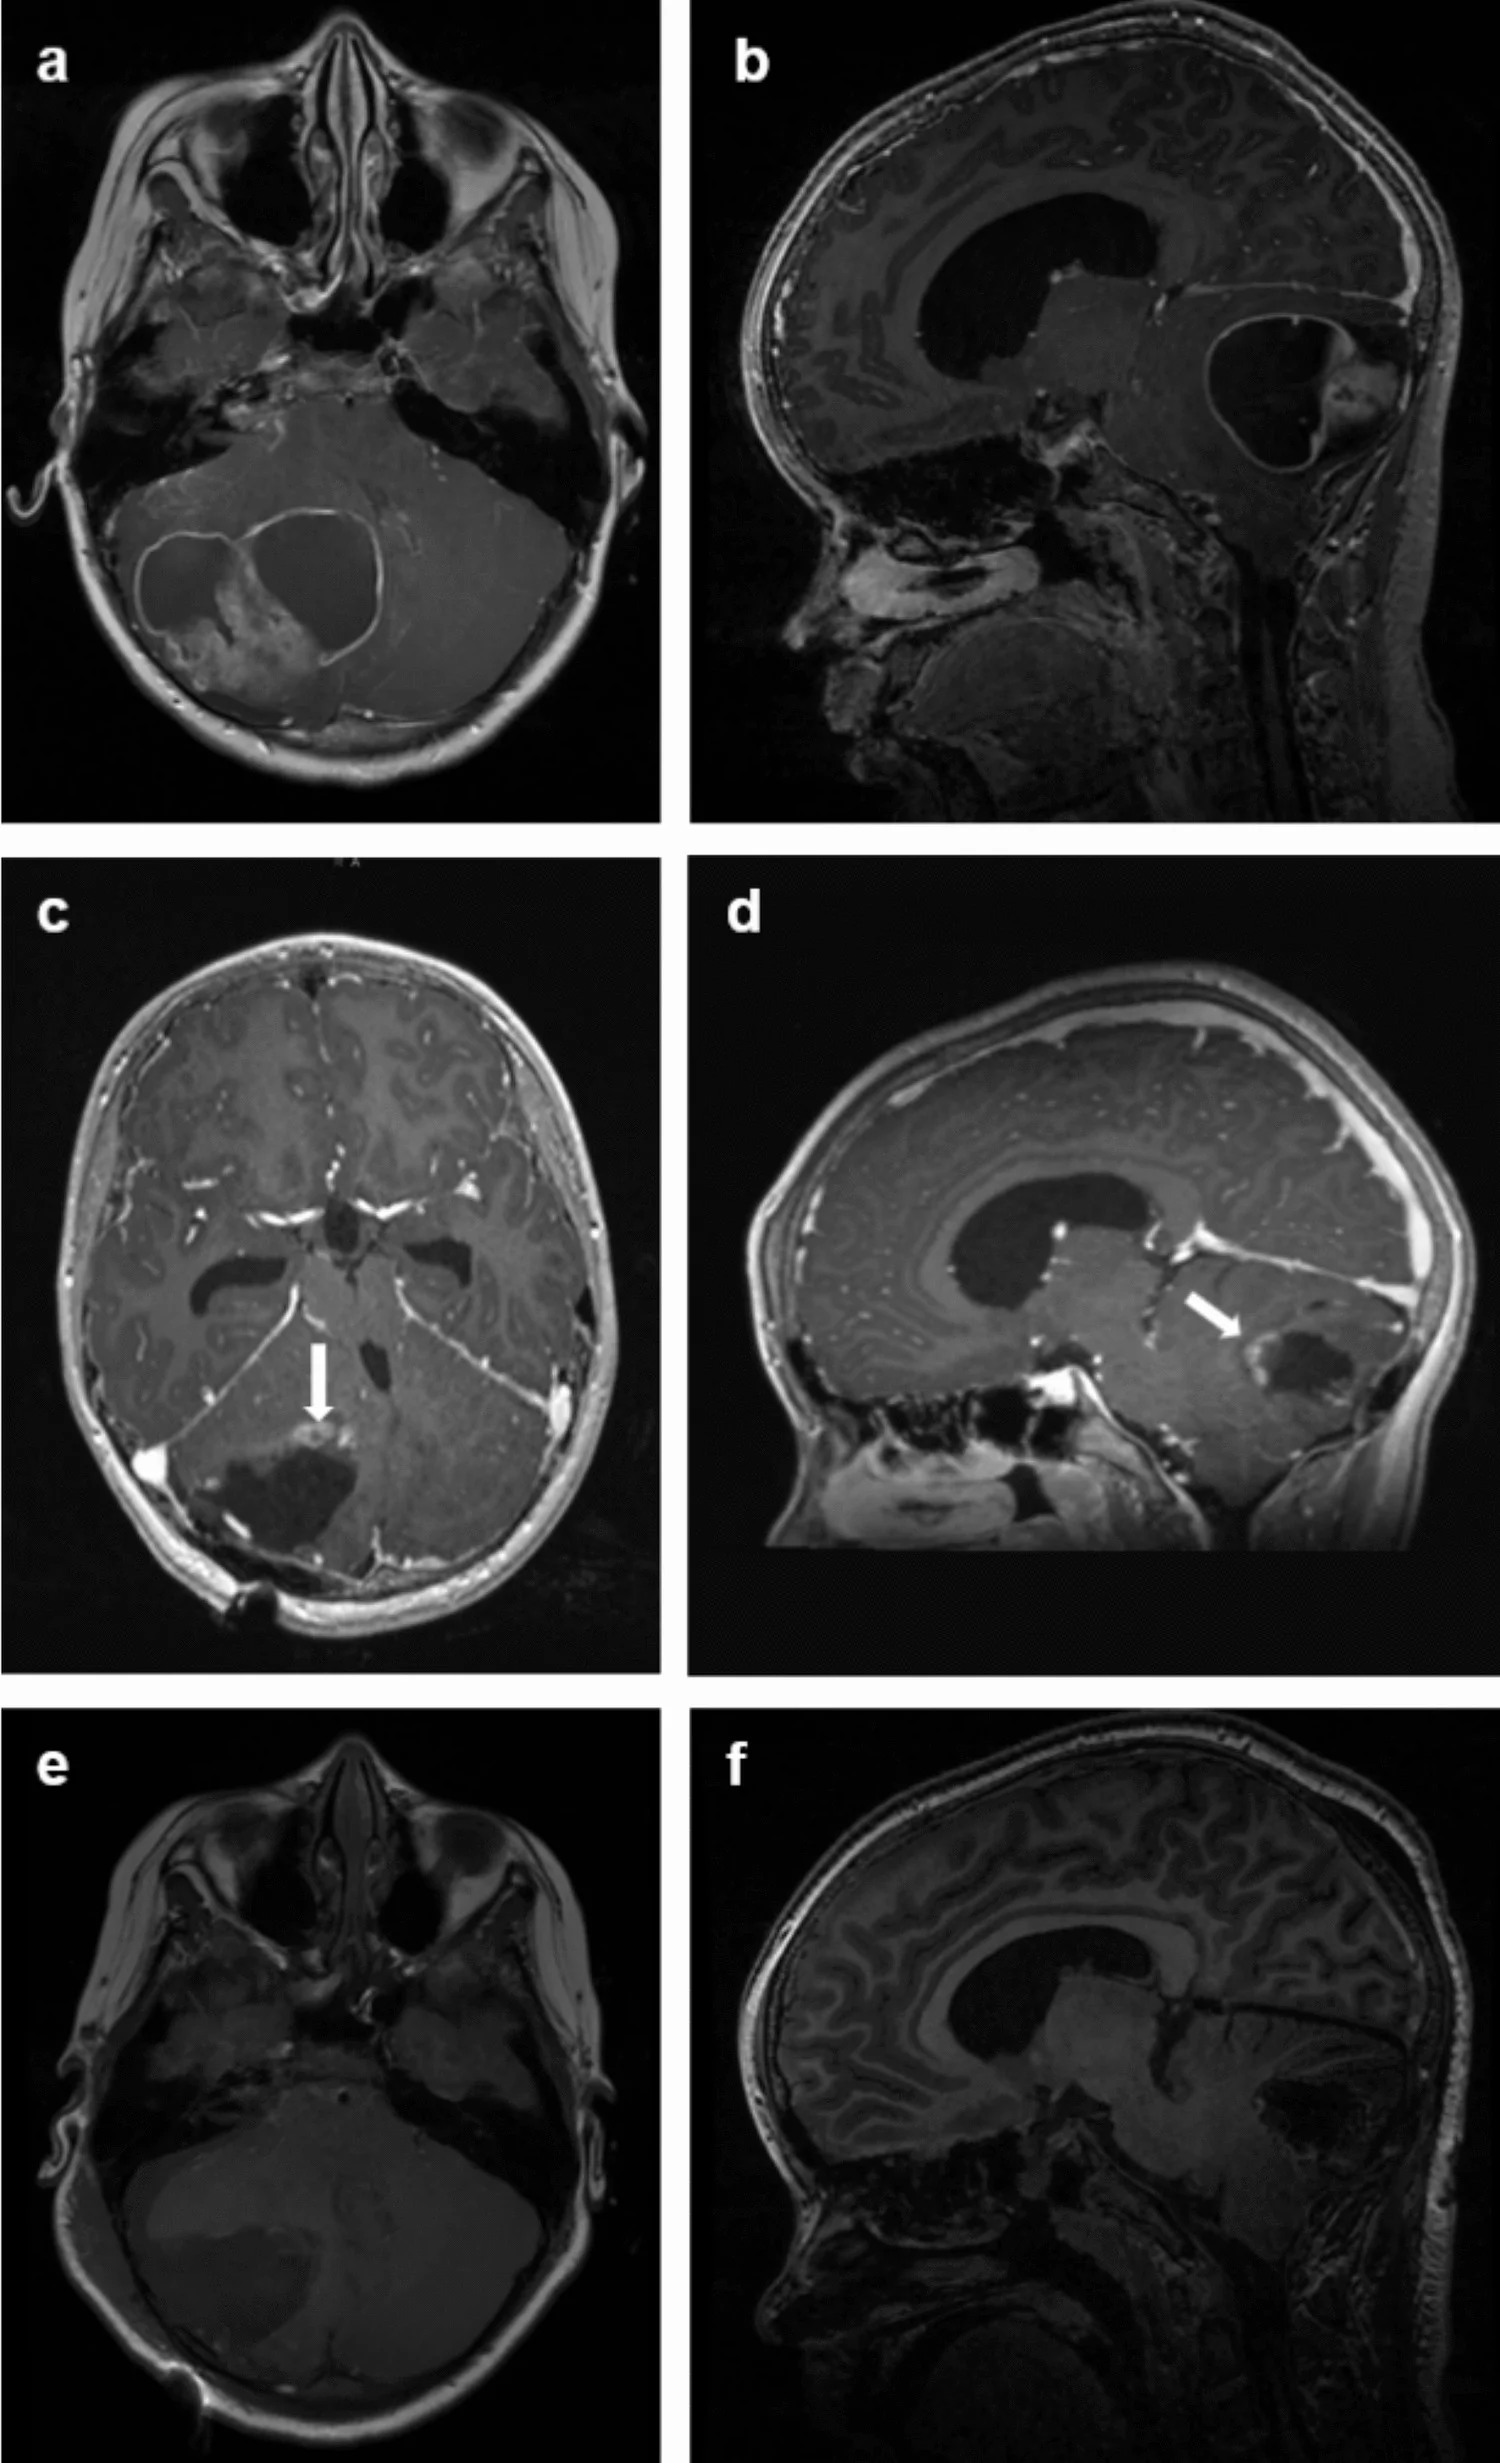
A 12-year-old patient presented with headaches, vertigo, and morning vomiting persisting over several weeks. Preoperative MRI (contrast-enhanced T1-weighted images, axial [a] and sagittal [b]) demonstrated a cystic mass in the right cerebellum with a solid, contrast-enhancing component, suggestive of a pilocytic astrocytoma. Gross total resection (GTR) was the intended extent of resection (EoR). Intraoperative MRI (c, d) revealed persistent contrast enhancement at the anterior–superior margin of the resection cavity (arrow), indicating residual tumor and demonstrating that only a subtotal resection (STR) had been achieved. Additional tumor resection was performed. Postoperative MRI (e, f), obtained the day after surgery, confirmed complete tumor removal, resulting in the final EoR of GTR
